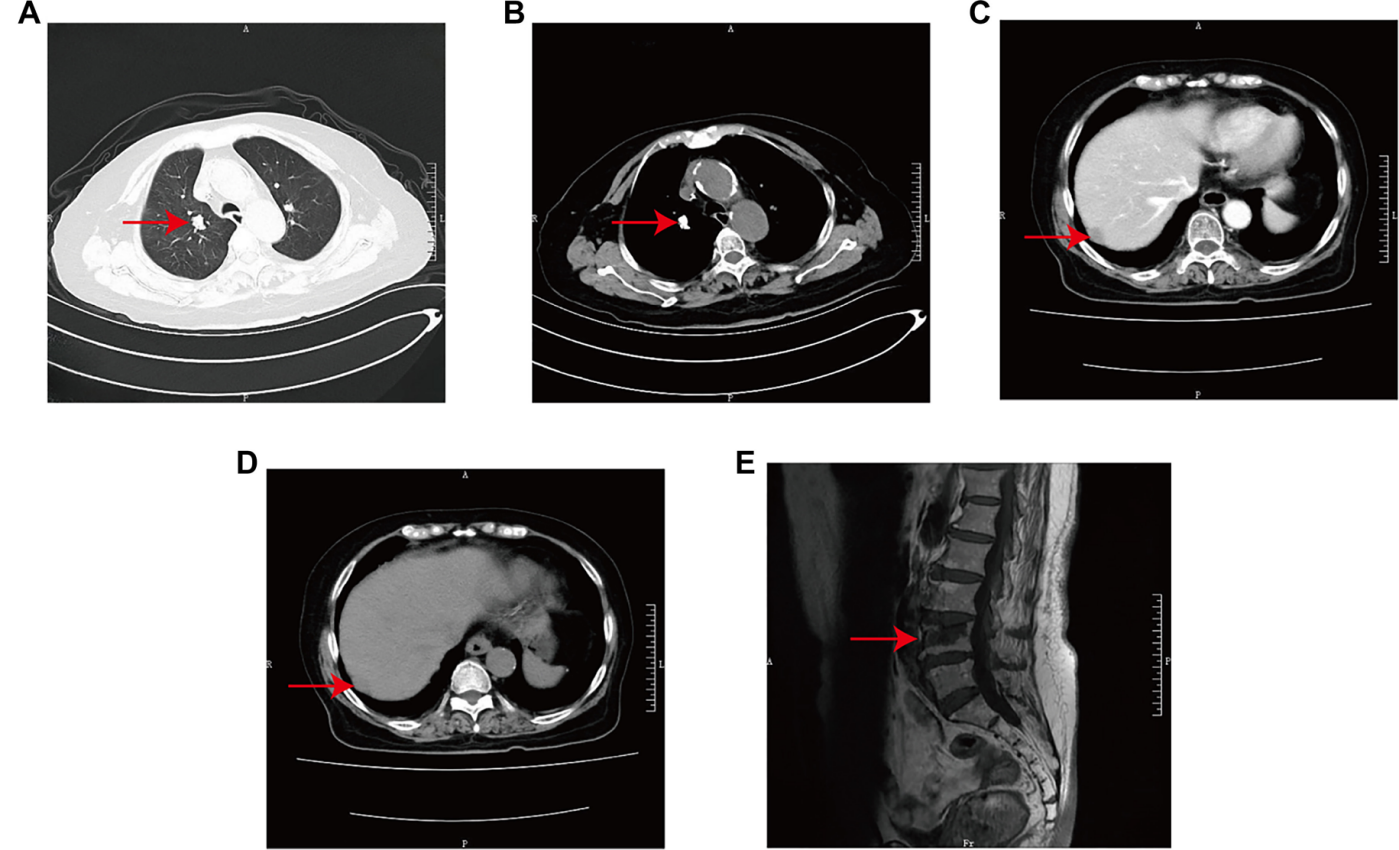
Radiographic data from case 3—CT and MRI images. These radiographic findings indicate the presence of metastases in the liver, lungs, and bones. (A) CT image using the lung window, showing lung metastasis (arrow). (B) CT image using the mediastinal window, also revealing lung metastasis (arrow). (C) Cross-sectional contrast-enhanced arterial phase CT of the abdomen, demonstrating liver metastasis (arrow). (D) Cross-sectional contrast-enhanced venous phase CT of the abdomen, further confirming liver metastasis (arrow). (E) Sagittal MRI images, highlighting vertebral metastasis (arrow). Abbreviations: CT: Computed tomography; MRI: magnetic resonance imaging.
